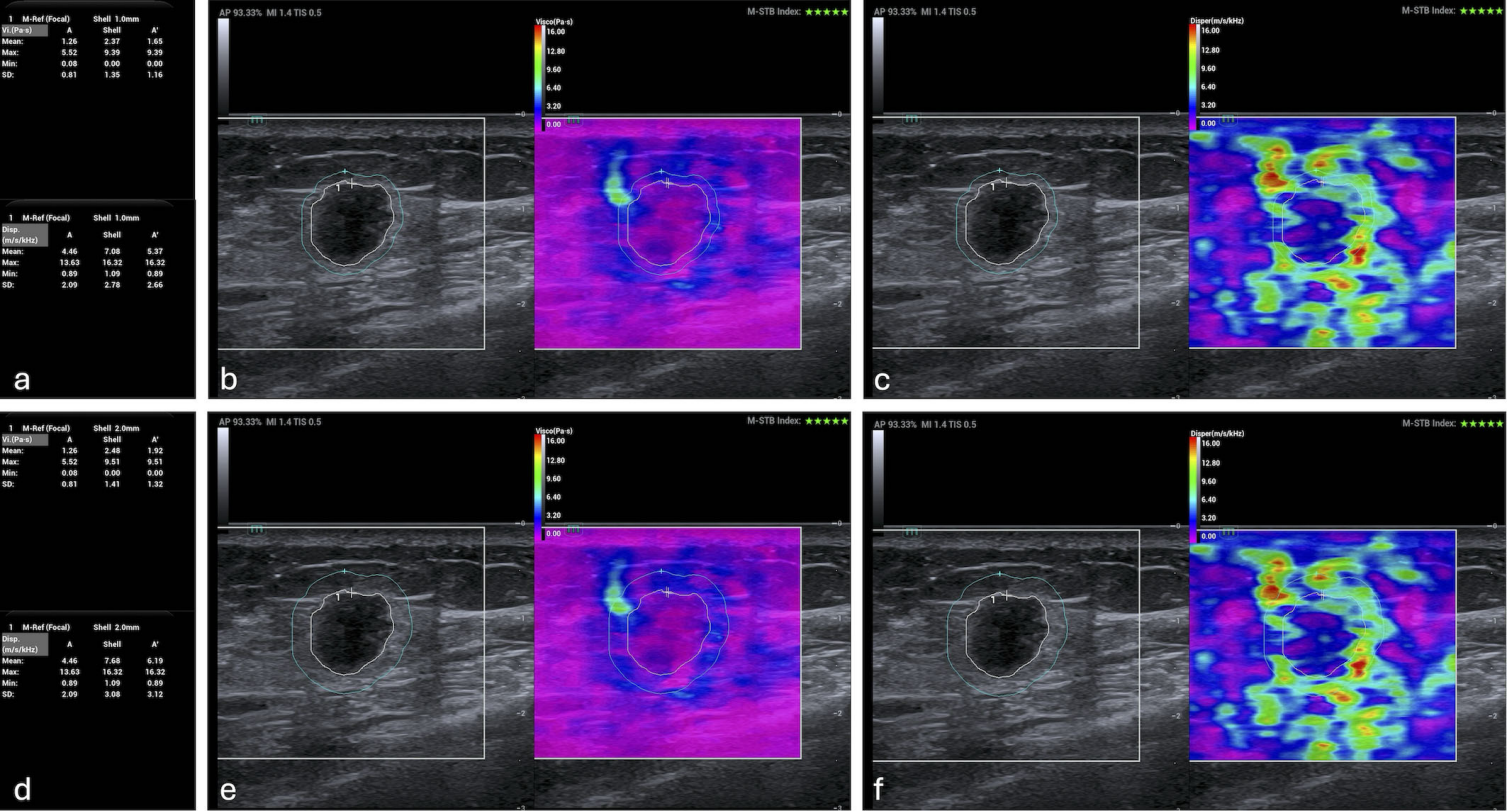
Representative ultrasound viscosity imaging analysis of a breast lesion. (a, d) Quantitative viscous parameter (VP) maps derived from the Voigt (units: Pa·s) and shear wave dispersion (SWD) (units: m/s/kHz) models, respectively. For each model, four statistical parameters (maximum, minimum, mean, and standard deviation) are displayed for three regions of interest: the lesion core (A), a 1-mm perilesional rim (Shell 1), and a 2-mm perilesional rim (Shell 2). (b, c, e, f) The corresponding grayscale ultrasound images underlaid with the VP distributions shown in panels a and d.
